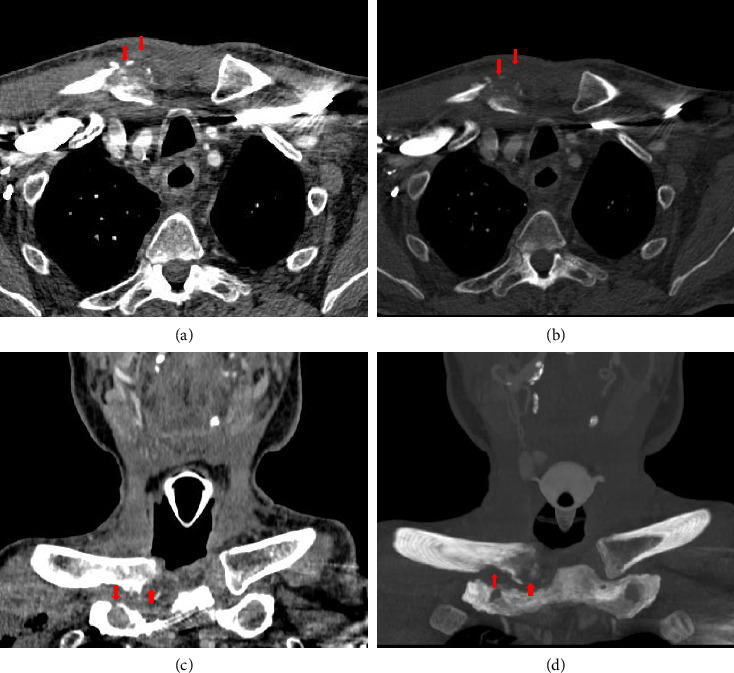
CT scan at 6 weeks later after clinical worsening: (a and b) axial and (c and d) coronal slides. Signs of sternoclavicular joint arthritis with enlargement of the bone erosions, osteolysis, and soft tissue augmentation (red arrows).
